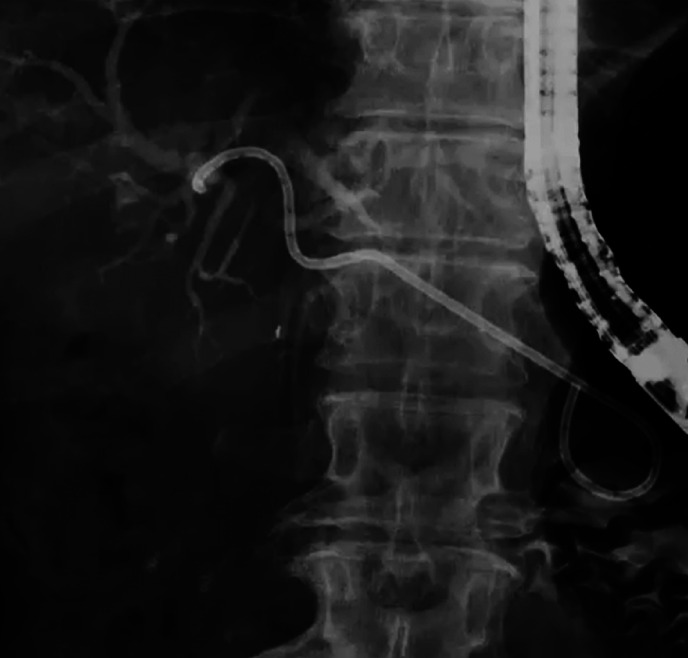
The spiral-shaped plastic stent was deployed as an endoscopic ultrasound-guided hepaticogastrostomy stent.
